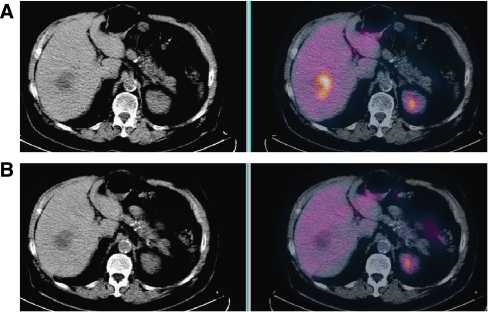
A liver metastasis from a gastric GIST before (A) and 1 week after (B) commencing imatinib therapy. FDG PET/CT scans, unenhanced CT (left), fused FDG PET and CT (right). Although there has been no morphological change in the metastasis, the abnormal baseline metabolic activity (colour scale) has rapidly resolved indicating sensitivity to the drug. The SUV fell from 5.0 to 1.8.
